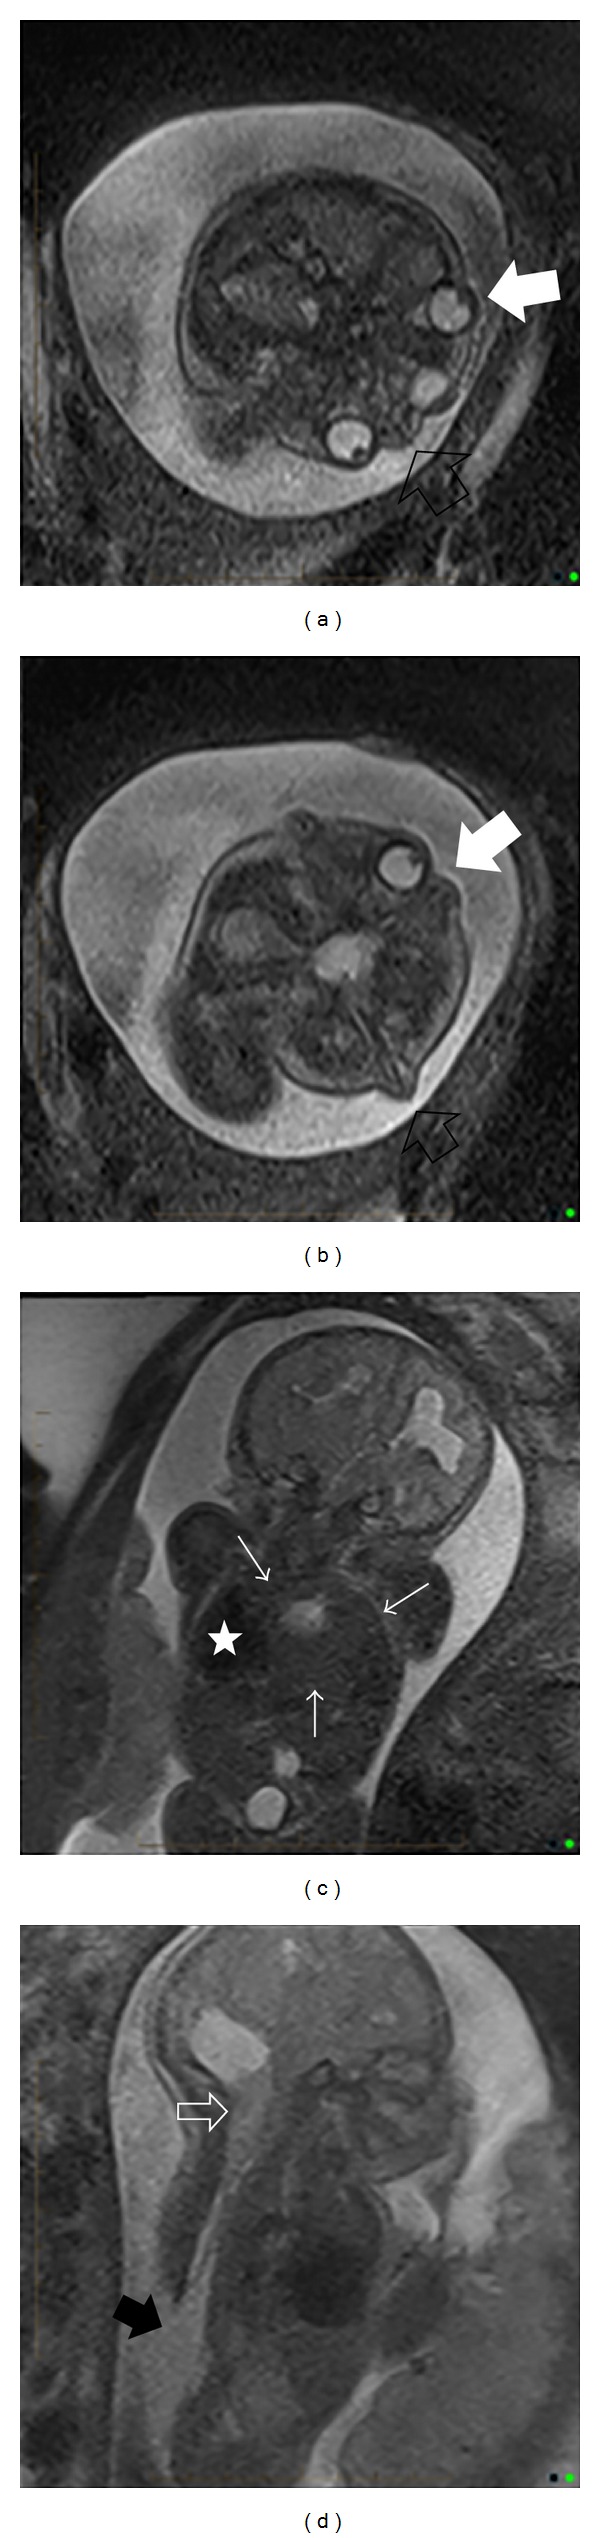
Axial images from fetal MRI (a and b) showing the left-sided (open black arrows) and right-sided (solid white arrows) fetal faces. Coronal image from fetal MRI (c) reveals a left-sided diaphragmatic hernia (thin white arrows) containing liver and bowel with displacement of the fetal heart (white star) to the right. Chiari II malformation with tonsillar herniation through the posterior foramen magnum (open white arrow) and associated lumbosacral spinal dysraphism (solid black arrow) is noted on a sagittal image (d).
